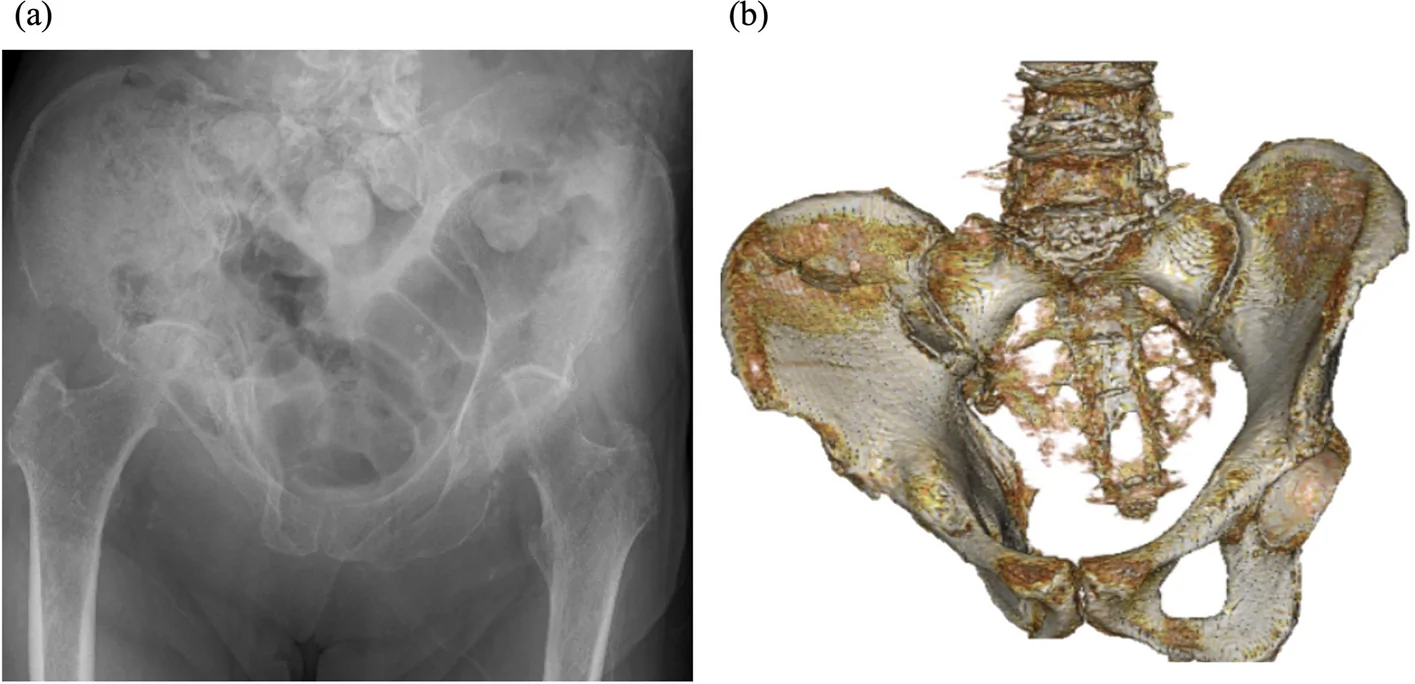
Original radiographic images. The (a) antero-posterior radiograph and (b) three-dimensional computed tomography scan images of a patient with Rommens type IIIa FFP were used to create QCT/FEA models
